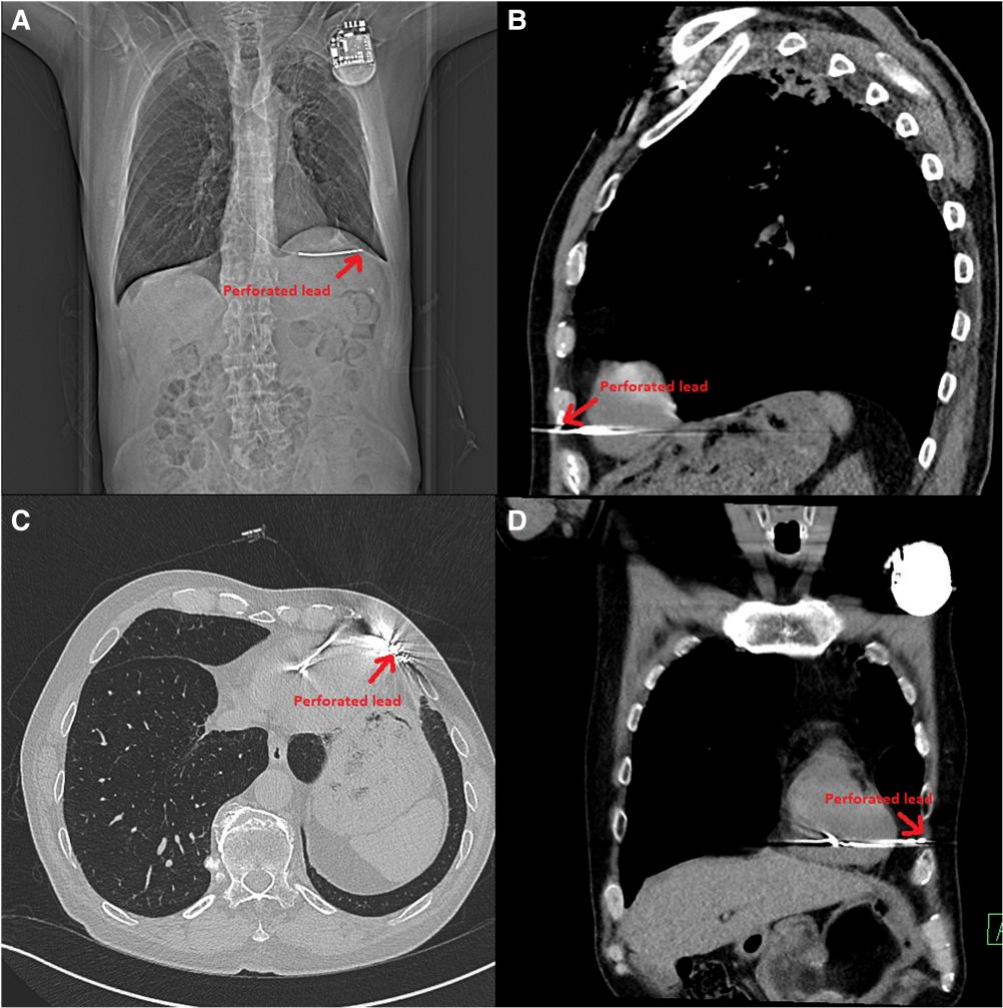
Computed tomography showing lead perforation through the pericardium to the intercostal muscle. (*A*) Initial topogram. (*B*) Sagittal view. (*C*) Transversal view. (*D*) Frontal view.
